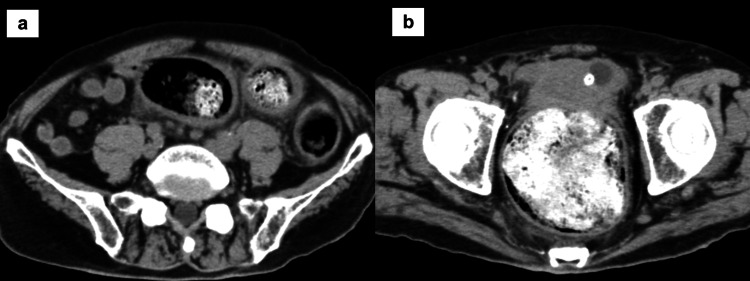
Abdominal computed tomography scan taken upon fever during hospitalization a) Sigmoid colon level. An increased concentration of peripheral adipose tissue is visible in the sigmoid colon. b) Rectum level. A significant amount of stool is confirmed in the rectum.
